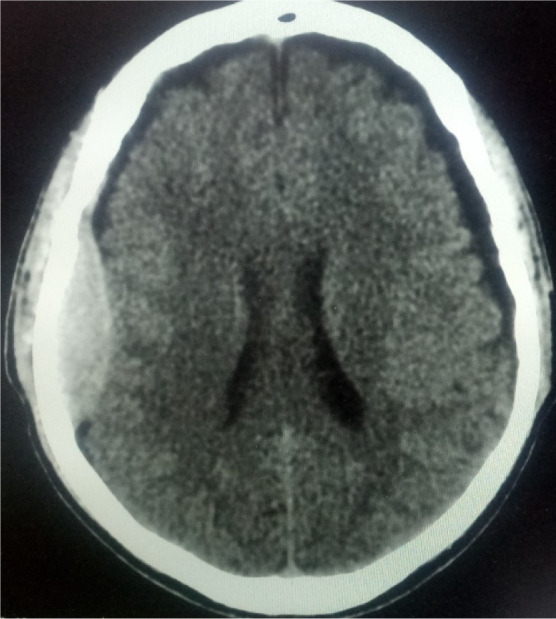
Axial non-contrast brain CT showing acute epidural haemorrhage in the right parietal region.
